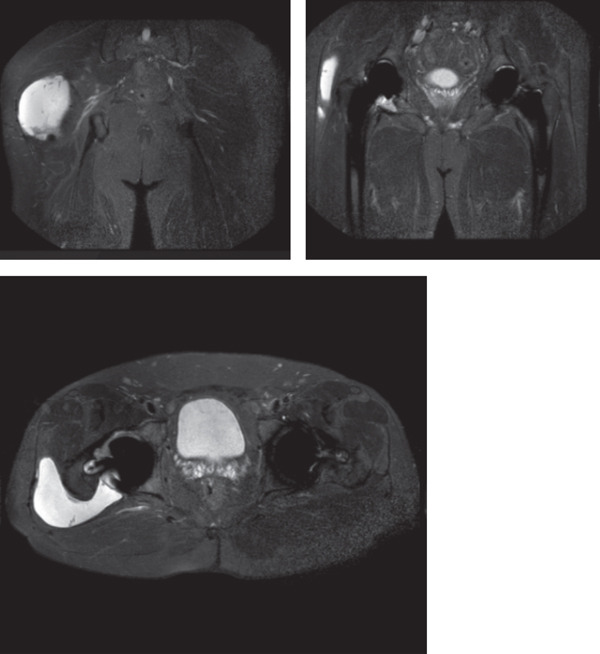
MRI of the right hip showing periprosthetic posterior fluid collection.
